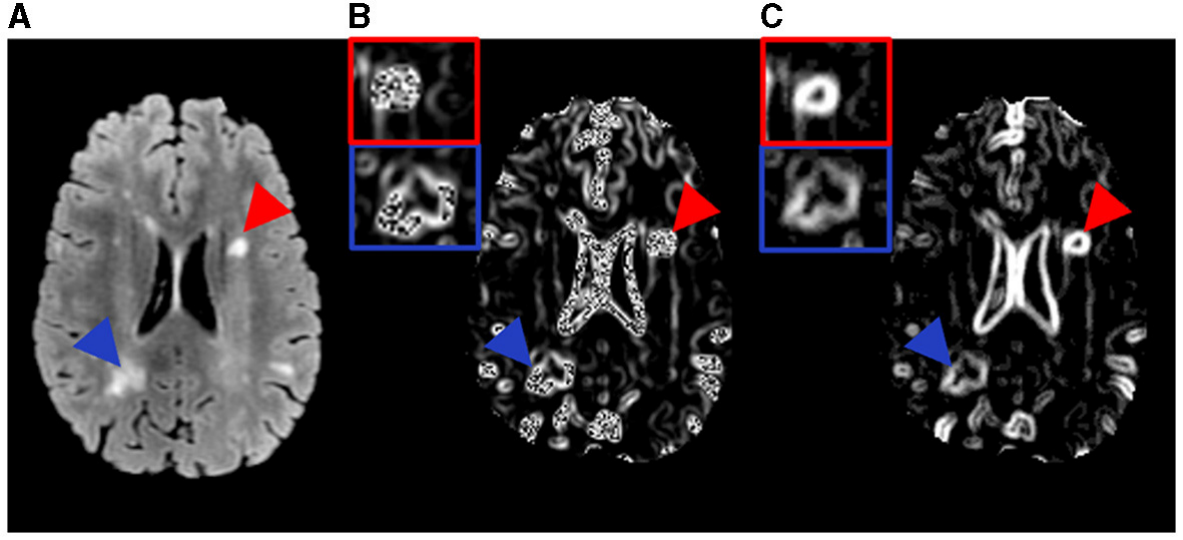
Example MR images and the texture feature maps from one RRMS participant examined in this study. Shown are a FLAIR image (A) as well as the resulting texture contrast (B) and texture dissimilarity (C) maps with corresponding lesions identified as severely demyelinated (red) or highly remyelinated (blue). Brighter signal indicates greater texture value and more heterogeneity in the maps. The participant has a disability score of 2.5 and disease duration of 17.1 years.
